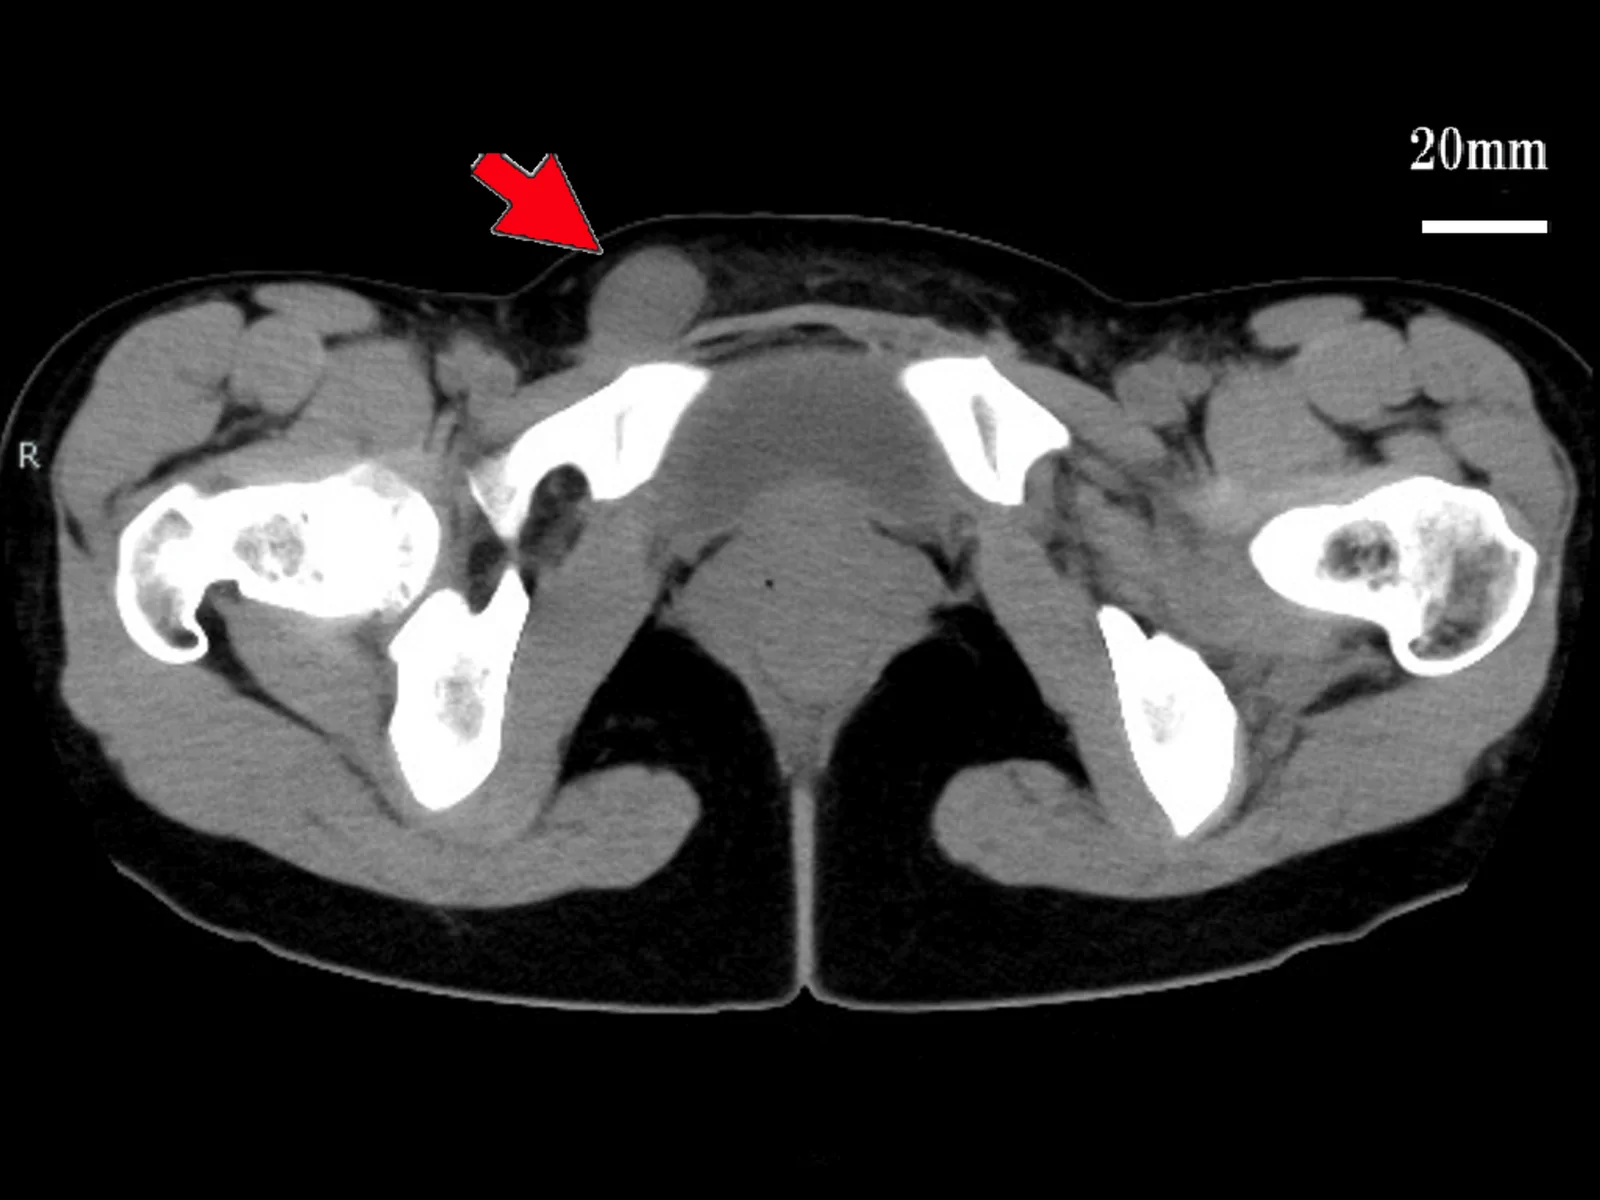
Plain pelvic computed tomography scan. A well-circumscribed 25-mm low-attenuation solid lesion is identified in the right inguinal region (arrow).
